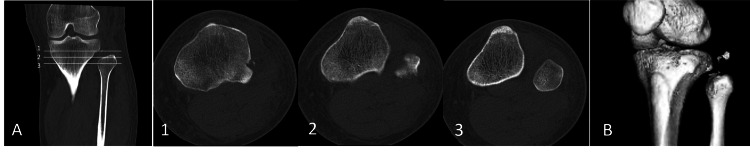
Computed tomography images (A) Computed tomography images of axial (1-3) and (B) 3D reconstruction images. The computed tomography image reveals a posterior lateral proximal tibiofibular dislocation with small bony fragments.
